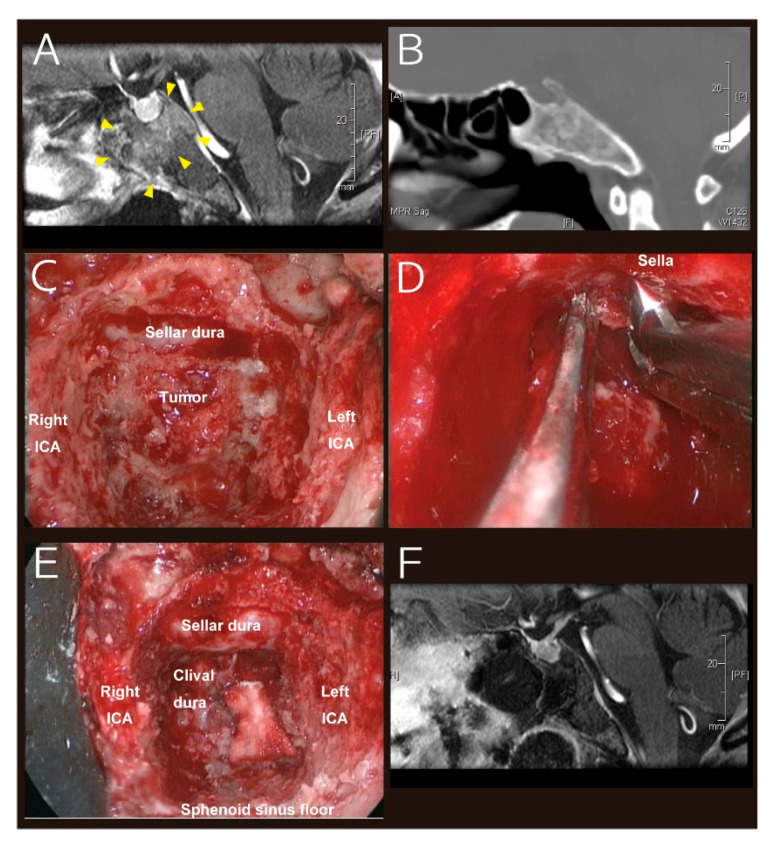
Patient #2. A 69-year-old woman presented with diplopia. MRI demonstrated an irregularly enhancing midline clival lesion ((A), contrast-enhanced T1-weighted image; yellow arrowheads indicate the mass). Of note, the patient showed a conchal-type sphenoid sinus ((B), computed tomography). An extended transsphenoidal transclival approach was selected. After opening the sphenoid sinus, plenty of clival bone had to be removed to expose the dura of the sellar floor and the tumor ((C), intraoperative image). Since the tumor was located under and behind the sella turcica, retraction of the sellar dura was necessary to remove the tumor ((D), intraoperative image). Gross total resection was achieved ((E), intraoperative image). MRI on POD 1 shows a good resection cavity with intact pituitary gland ((F), contrast-enhanced T1-weighted image). Pathological examination revealed chordoma. Sodium levels demonstrated a mild increase in phases 1 and 2, then dropped to 123 mmol/L on POD 10 when the patient developed severe fatigue. Sodium level improved to 134 mmol/L on POD 12 only with oral salt supplementation and mild fluid restriction, and the symptoms resolved accordingly.
